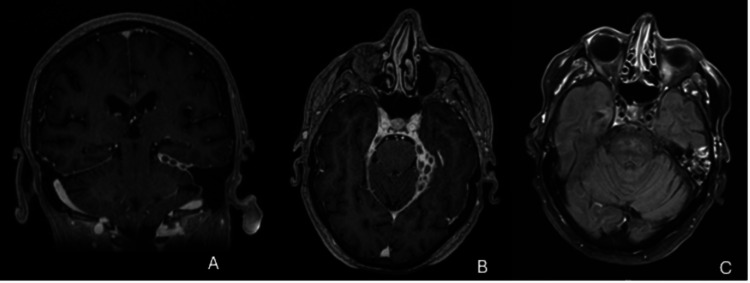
Coronal and axial navigation protocol cranioencephalic MRI (A/B) Coronal and axial navigation protocol cranioencephalic MRI showing a contrast-enhancing extra-axial lesion in the left cerebellar tentorium initially suspected as a multiloculated meningioma. (C) Axial T2-FLAIR cranioencephalic MRI highlights atrophic-gliotic changes in the left temporal region, interpreted as post-surgical sequelae. These findings were critical in ruling out alternative causes and identifying surgical targets.
